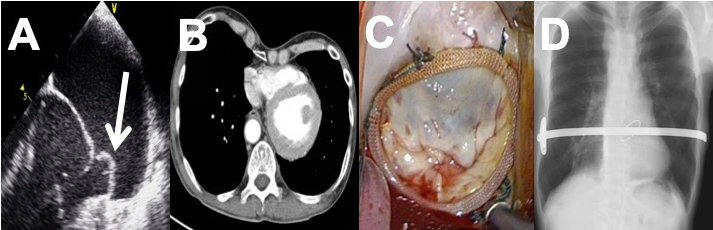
Perioperative and Procedural Images of Staged Endoscopic Mitral Valve Repair and Pectus Excavatum Correction Surgery A: Trans-thoracic echocardiography indicating a prolapsing P2 mitral valve segment (white arrow). B: Computerized tomography indicating cardiac displacement to the left hemithorax. The Haller Index* and the Correction Index^†^ were calculated to be 3.1 and 33.9%, respectively. C: Endoscopic mitral valve repair. D: Post-operative chest X-ray indicating satisfactory Nuss bar positioning. *Haller Index: the ratio of the transverse thoracic (25.4 cm) and minimum sternovertebral diameter (7.6 cm). ^†^Correction Index: the indentation depth (3.9 cm) as a percentage of the maximum sternovertebral diameter (11.5 cm).
